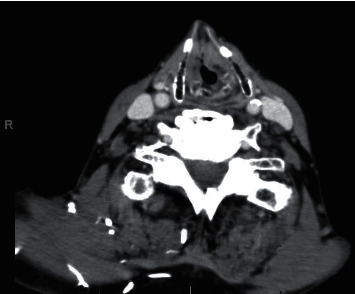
Axial CT neck with contrast demonstrating fullness of the left glottis.
